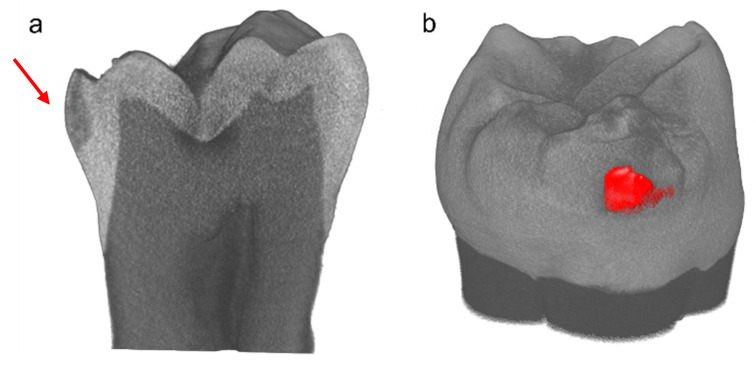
(a) Sagittal cross-section image of reconstructed µ-CT image of the representative sample. WLS appeared as a slightly darker triangular-shaped region on the left side of the image (red arrow). (b) The red color was used to better highlight the differences in MD.
